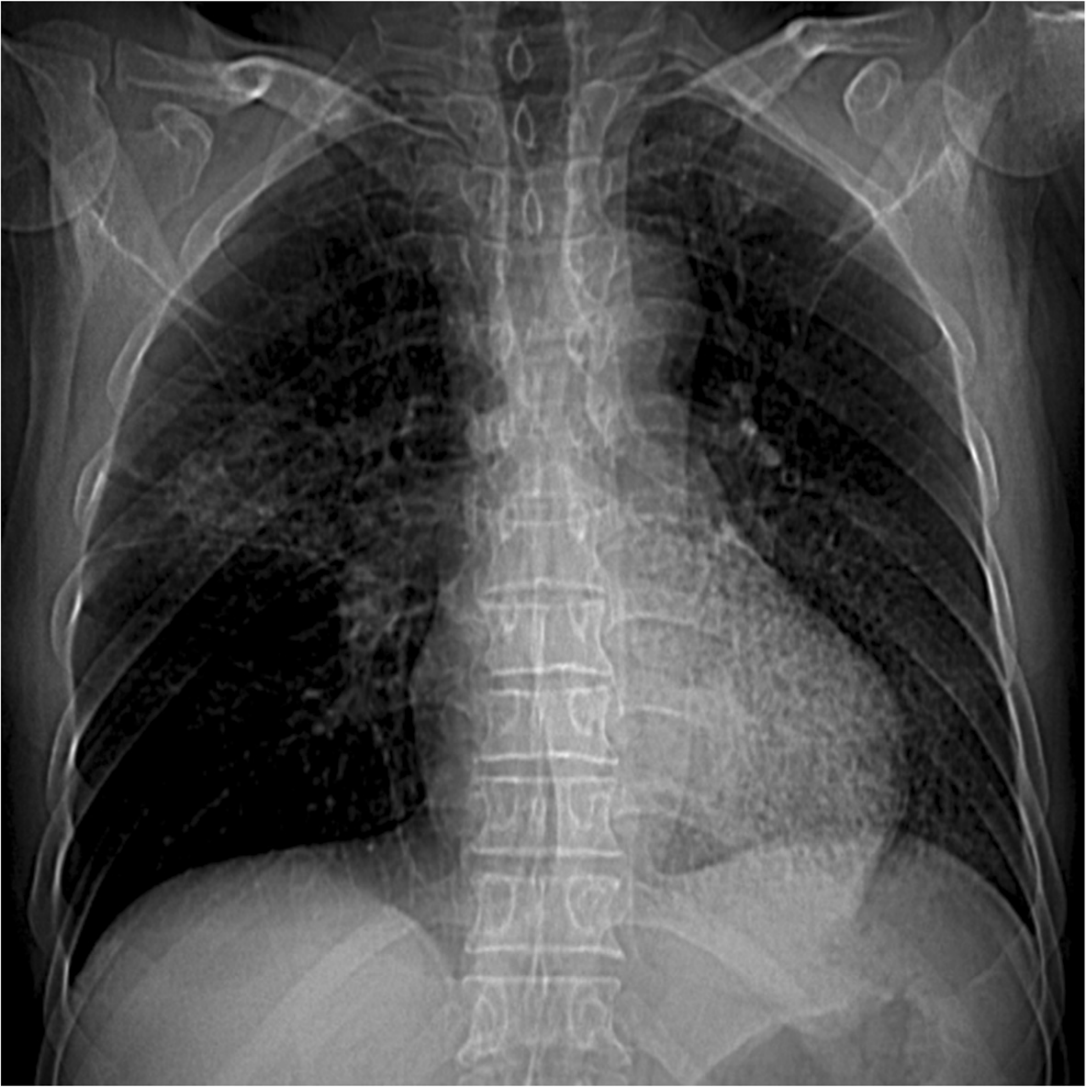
Chest imaging. Chest X-ray shows multiple bilateral nodules with surrounding bilateral reticular fibrotic lesions
